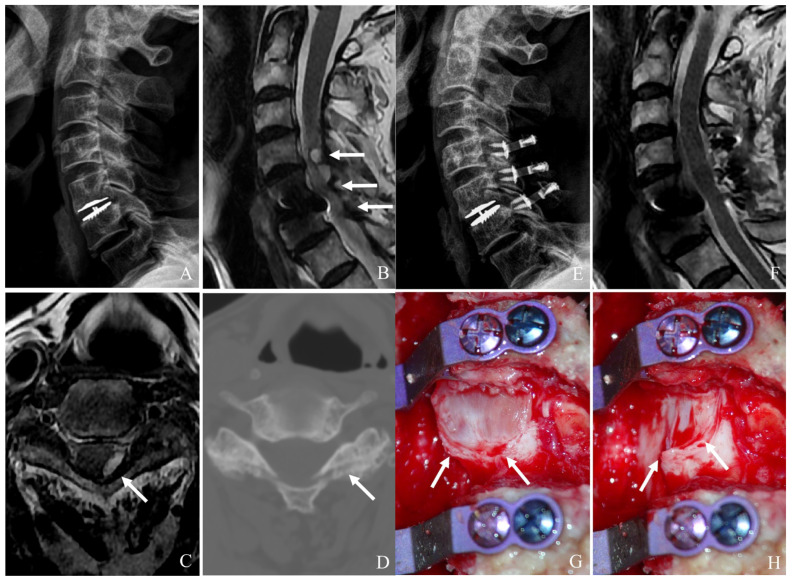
Plain X-rays (A), magnetic resonance image (B,C), computed tomography (D), and clinical photo (G) showing hypertrophy of ligamentum flavum at C4–6 and facet cyst at C4–5 causing cord compression (white arrows). The patient underwent C4accept–6 open-door laminoplasty and removal of facet cyst, leading to complete cord decompression (E,F,H).
